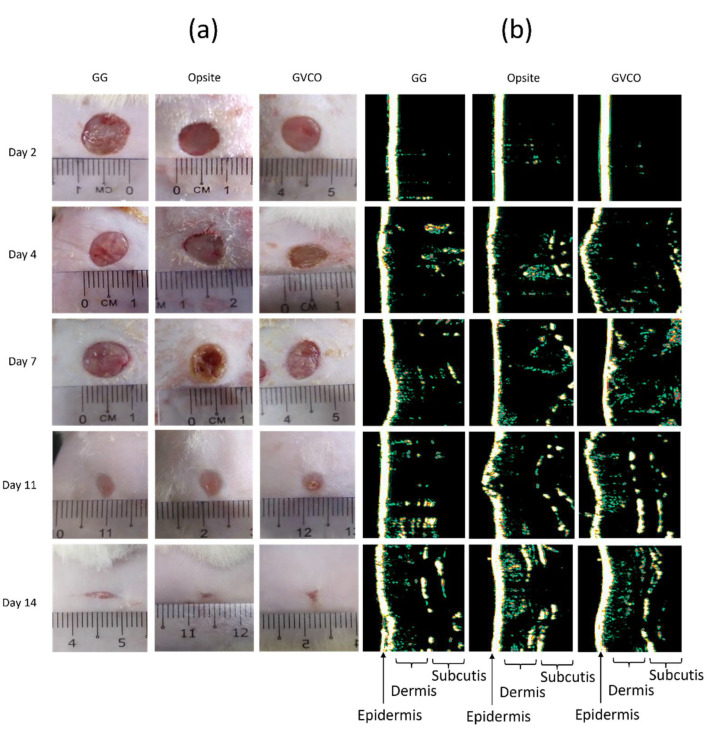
(a) Wound healing studies and (b) typical ultrasound images of wound skin on gellan gum (GG), Opsite, and GVCO80 hydrogels on days 2, 4, 7, 11, and 14.
